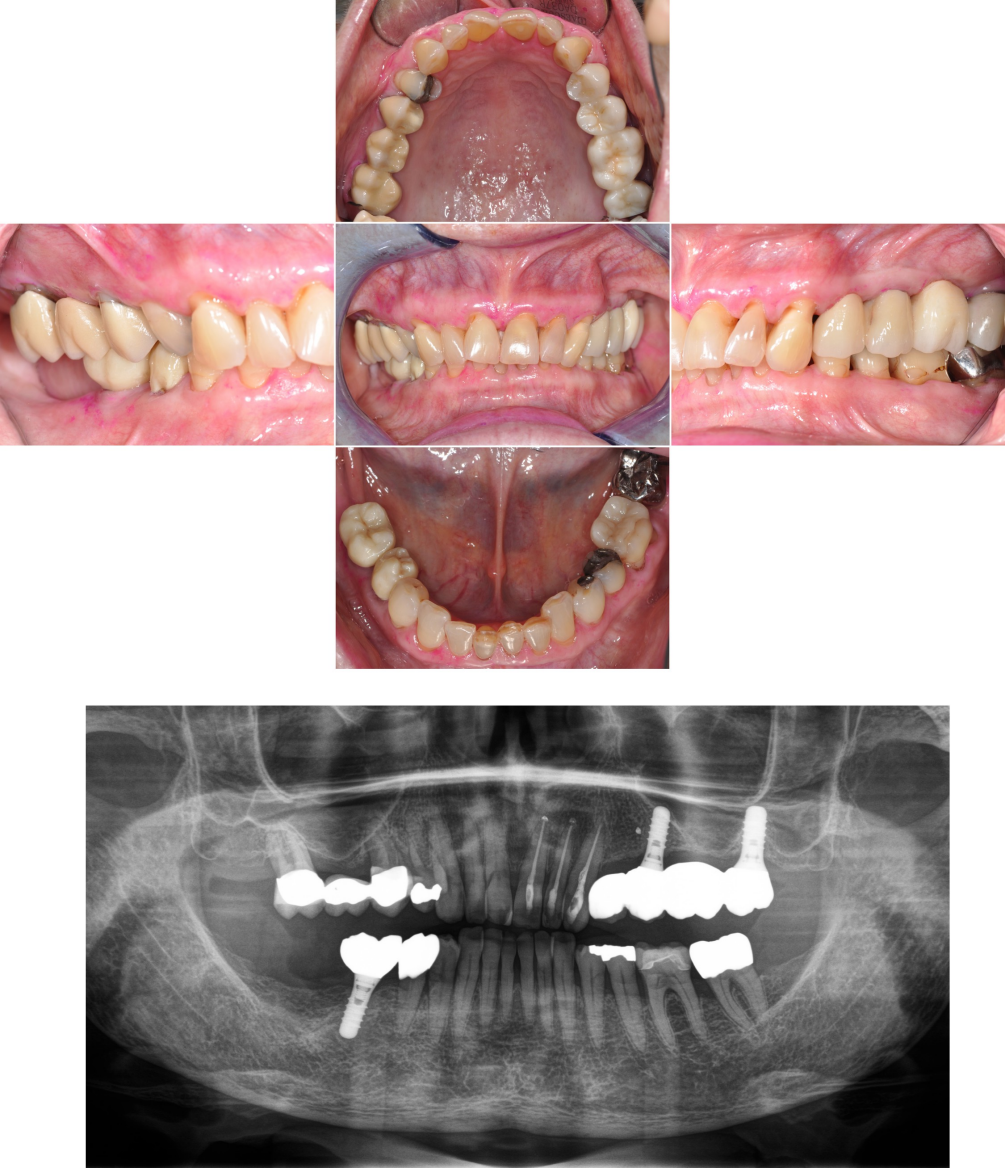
Exemplary intraoral digital photography status (A) and corresponding panoramic radiograph (B).
